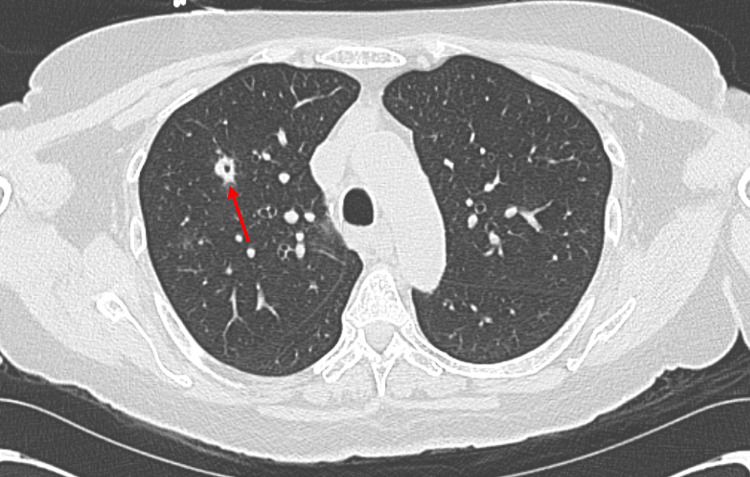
CT scan of the chest with IV contrast showcases a cavitary pulmonary nodule (red arrow).
